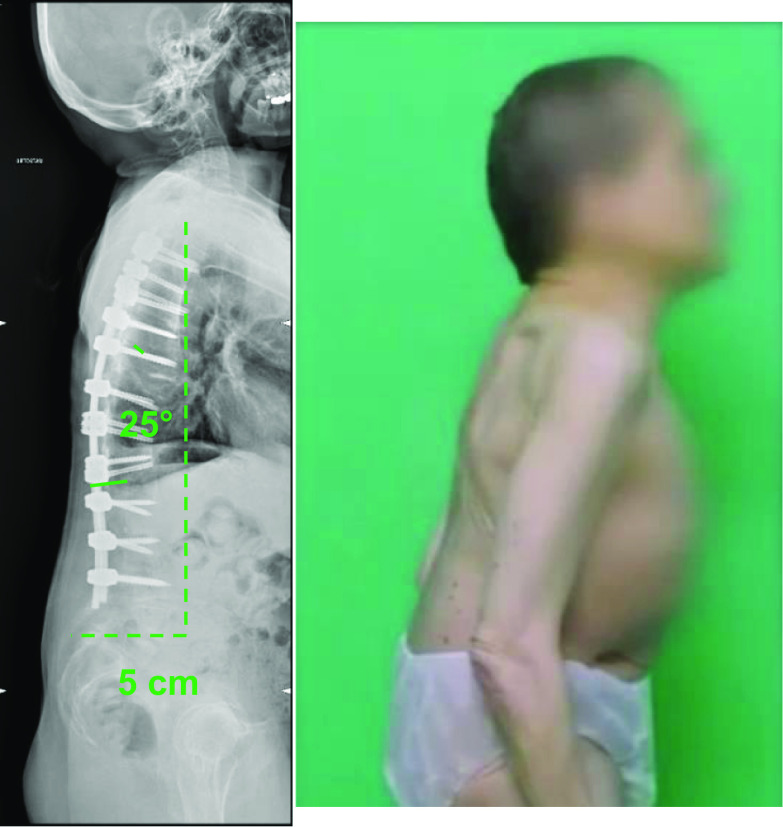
Case 1: postoperative radiographic and clinical presentation
